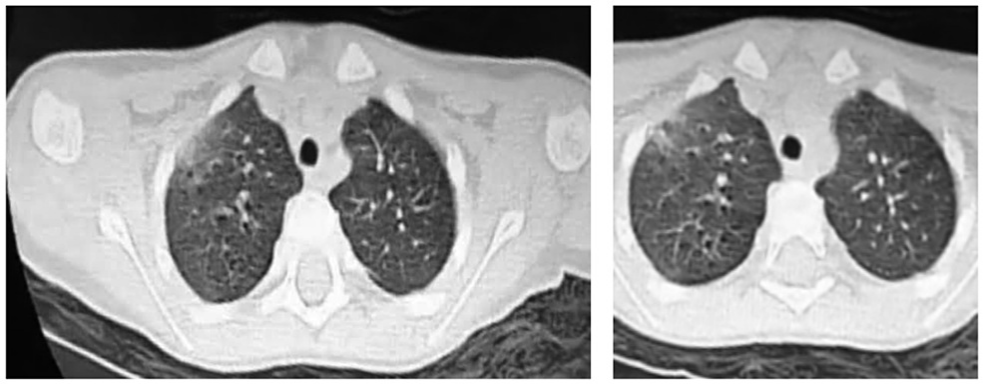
Axial images of the present case’s chest CT showing ground-glass opacities with consolidations
